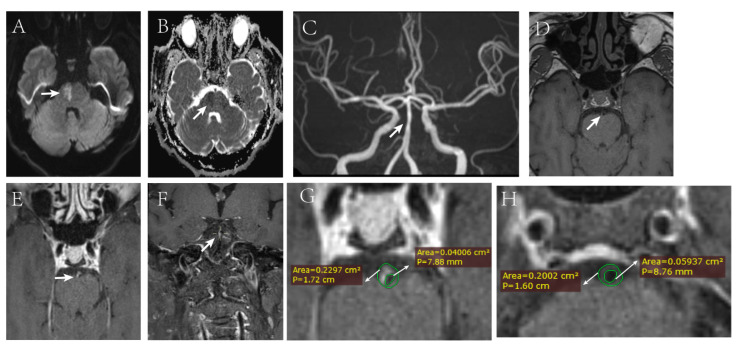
A 57-year-old male patient presented with dizziness accompanied by left limb weakness for 1 day. DWI and ADC showed limited diffusion in the right brainstem (A,B). MRA showed there was a mild stenosis in basilar artery (C). The 3D-T1-SPACE images perpendicular to the long axis of the vessel wall shows the irregular plaque in the ventral and right side of the vessel wall before enhancement (D), and the plaque is obviously strengthened after enhancement (E,F). The vessel area (VA) and lumen area (LA) were measured at the maximal lumen narrowing (MLN) and reference sites (G,H). The remodeling index (RI) was calculated as 0.2297/0.2002(1.147), the result was ≥ 1.05, so it was regarded as positive remodeling. Wall area (WA) was measured using VA–LA, WA_MLN_ = 0.1897 (0.2297 − 0.040), WAreference = 0.1408 (0.2002 − 0.0594). Plaque burden was calculated as 0.1897/0.2297 (0.826), and percent luminal area stenosis was calculated as (1 − 0.040/0.059) × 100% (32.2%). DWI, diffusion-weighted image; ADC, apparent diffusion coefficient.
